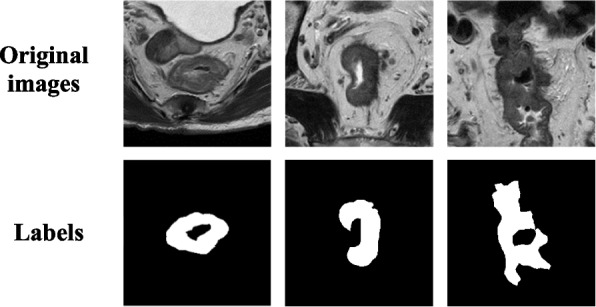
Typical examples of MR imaging of rectal cancer. Each MRI image of rectal cancer was from a different patient, and it was evident that the morphology of different rectal cancers varied greatly
